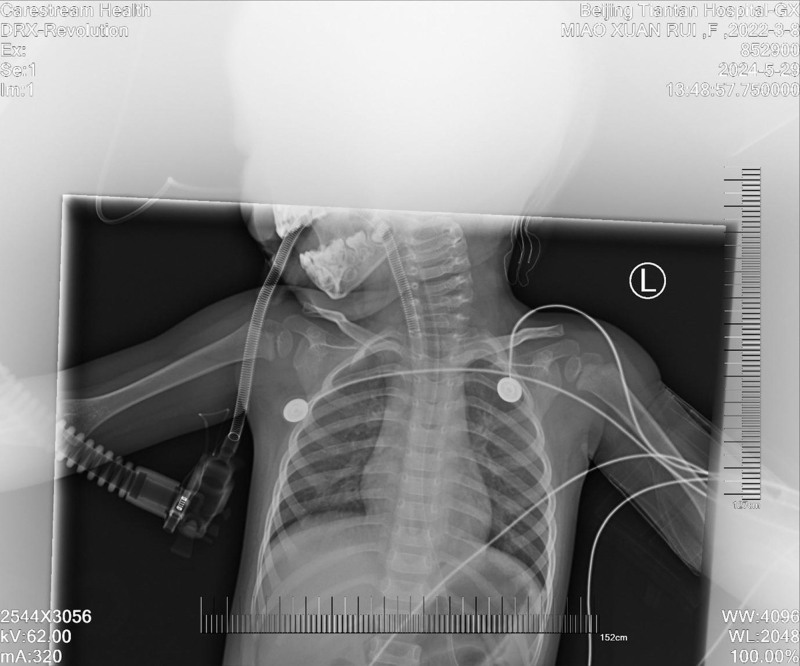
Postoperative chest X-ray image showing resolution of pulmonary edema in a 2-year-old patient after suboccipital craniotomy. X-ray imaging revealed the absence of pleural effusion and clear pulmonary fields, demonstrating successful treatment of acute NPE. NPE = neurogenic pulmonary edema.
